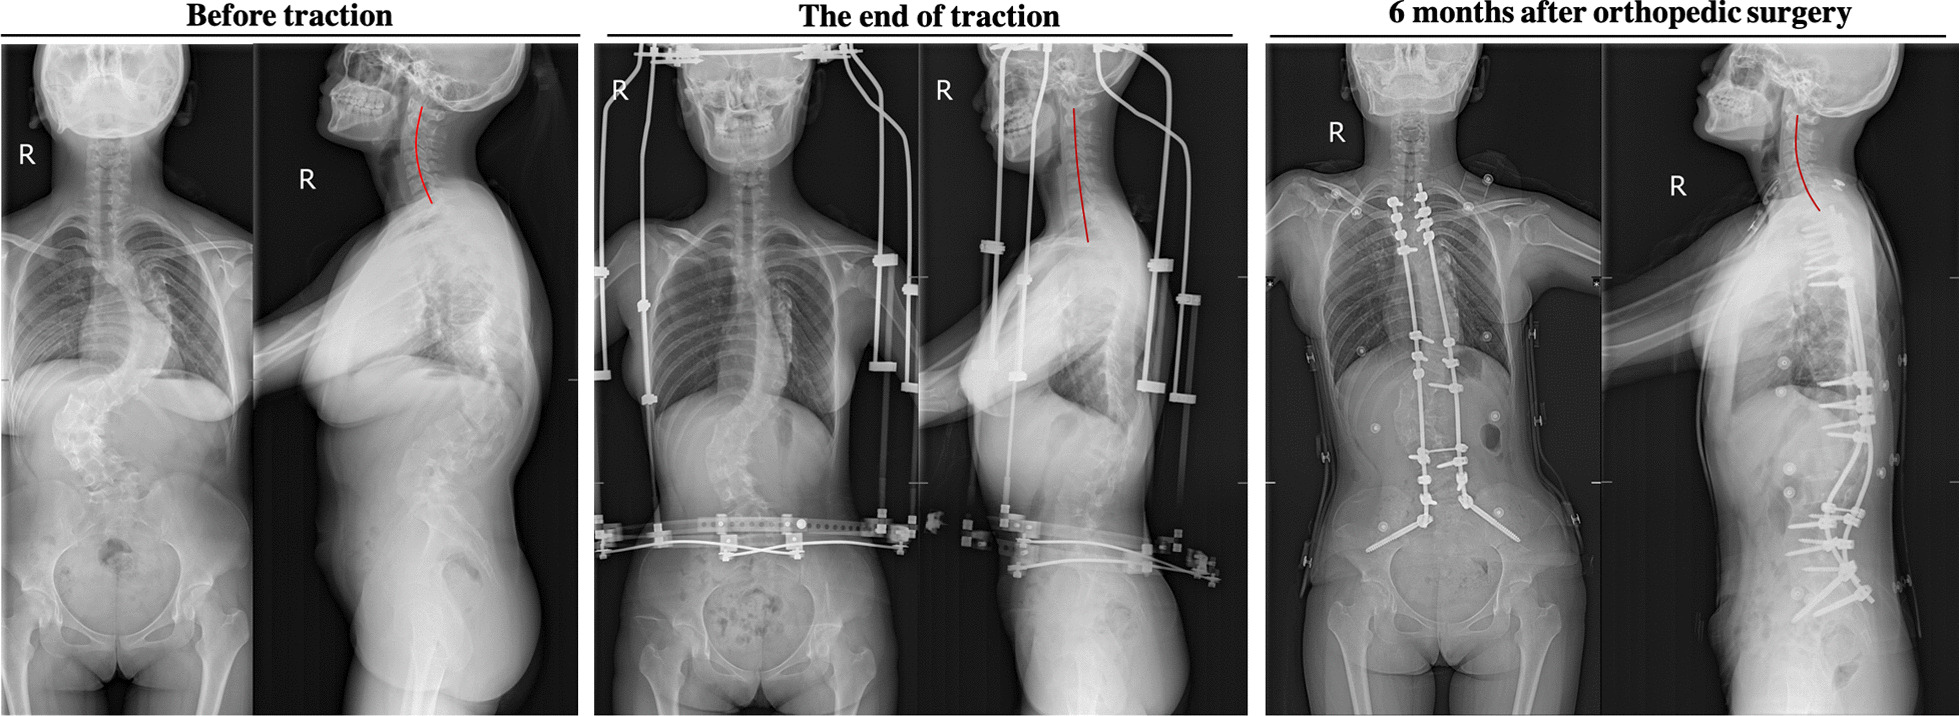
A 17-year-old female, with a height of 142 cm and a weight of 42.5 kg, was diagnosed as severe rigid spinal deformity. The operation time was 55 min, intraoperative blood loss was about 100 ml, and the total traction duration was 64 days. After traction, the height was 148 cm and the total traction volume was 7 cm. X-ray examination also showed that the cervical curvature was significantly straightened after Halo-pelvic fixation brace traction, and the cervical spine sagittal alignment was significantly recovered after removal of the fixation brace
